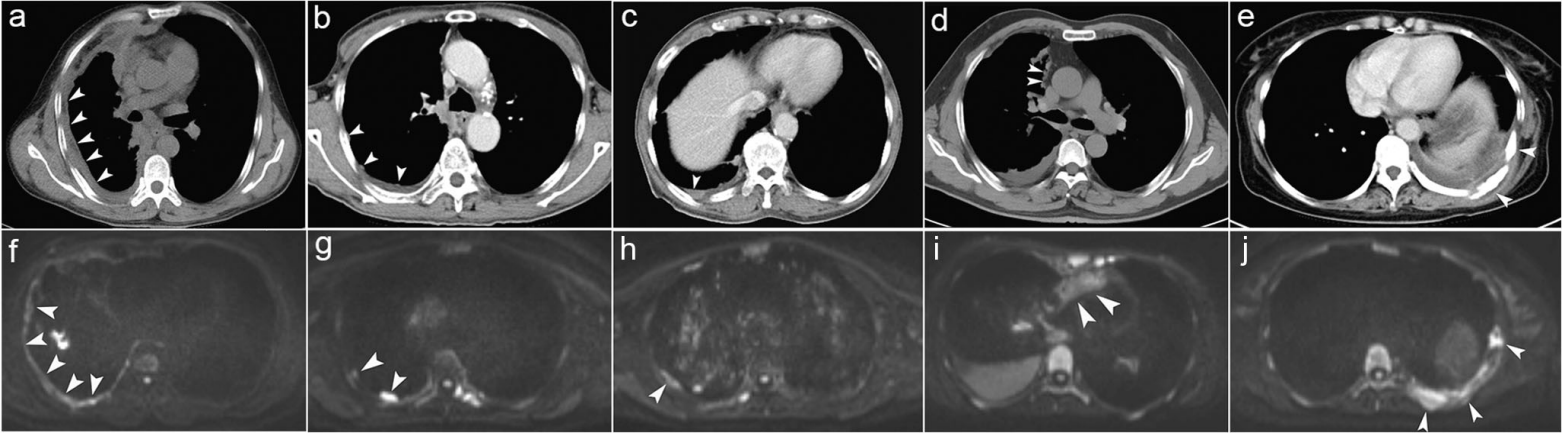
Representative pleural malignant features on CT (a–e) and DWI (f–j) are marked by the white arrow: a circumferential pleural thickening; b nodular pleural thickening; c visceral pleural thickening > 1 cm; d mediastinal pleural involvement; e chest wall invasion and rib destruction at multiple sites; f circumferential pleural thickening; g nodular pleural thickening; h thready pleural thickening; i mediastinal pleural involvement; j chest wall invasion and rib destruction at multiple sites
